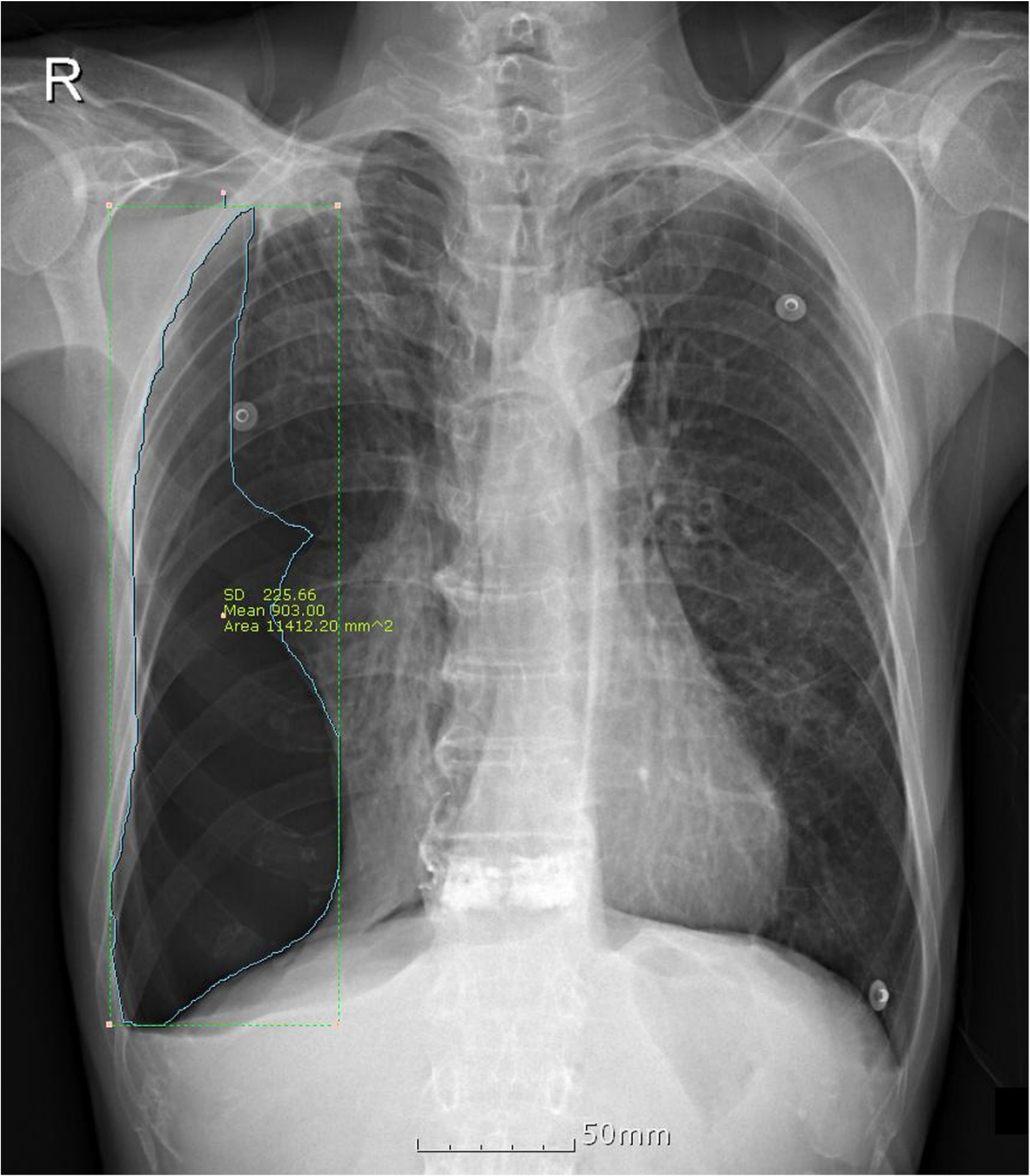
Measuring the size of pneumothorax. The area of pneumothorax were measured on a chest radiograph using an automated region of interest caculator in a picture archiving communication system.
